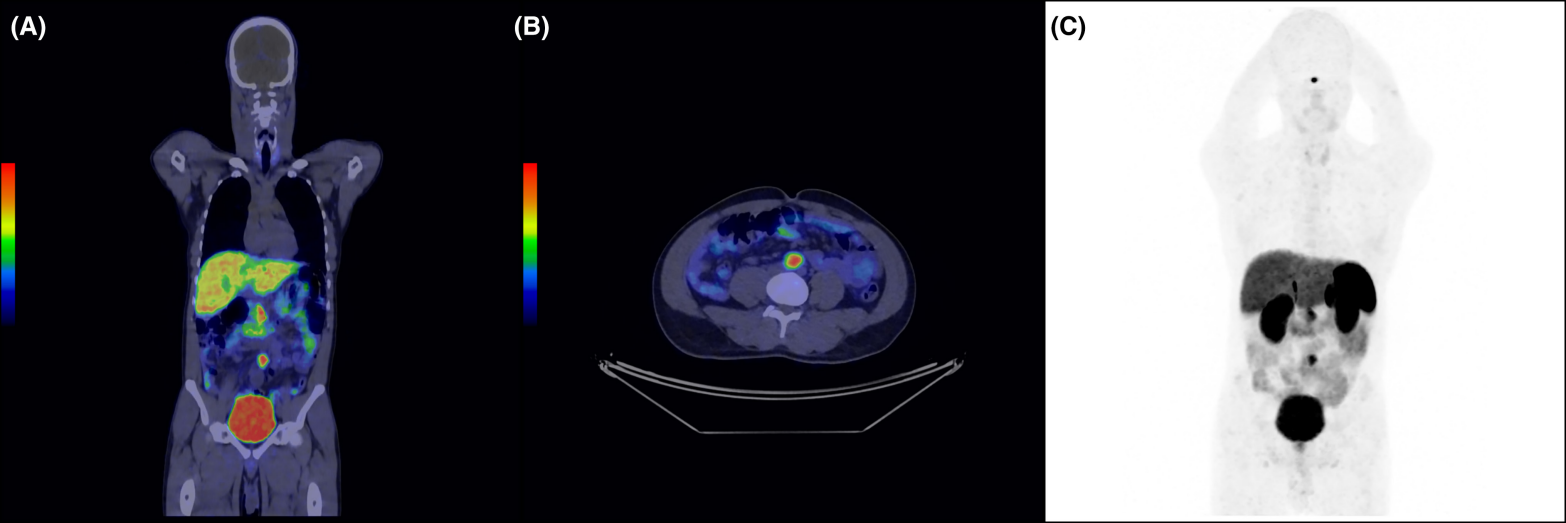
Coronal (A), axial (B), and MIP (C) views of the DOTATATE PET, showing significant radiotracer uptake (SUVmax 10.8) within the 23 × 33 mm mass located at the aortic bifurcation. MIP: Maximum intensity projection, PET: positron emission tomography, SUVmax: maximum standardized uptake value.
